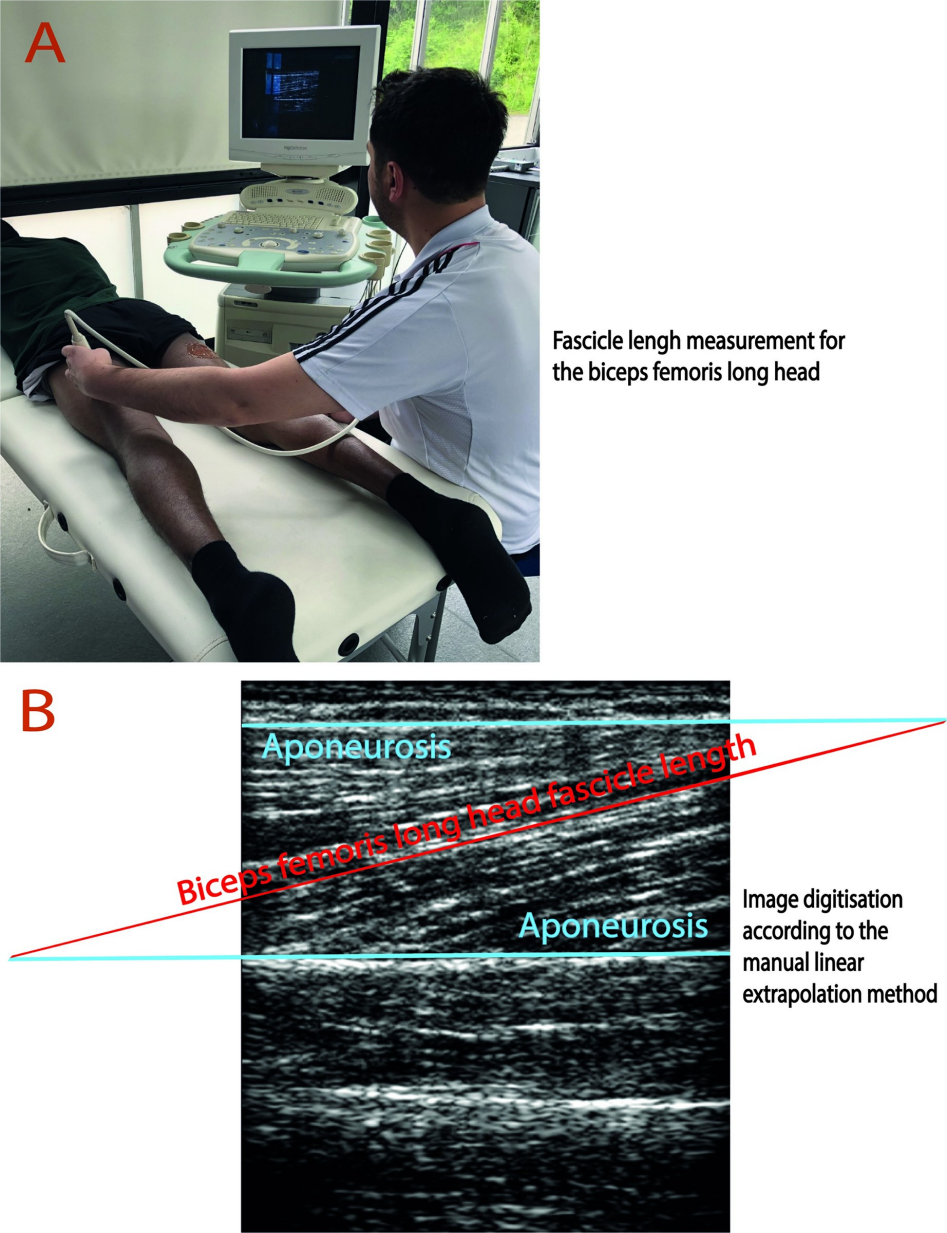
The biceps femoris long head fascicle length measurement and digitisation. A: 2-dimensional ultrasound images were taken from the mid-thigh for both legs. B: Digitisation for the biceps femoris long head fascicle length according to the manual linear extrapolation method. The individual pictured in Fig 2 has provided written informed consent (as outlined in PLOS consent form) to publish their image alongside the manuscript.
